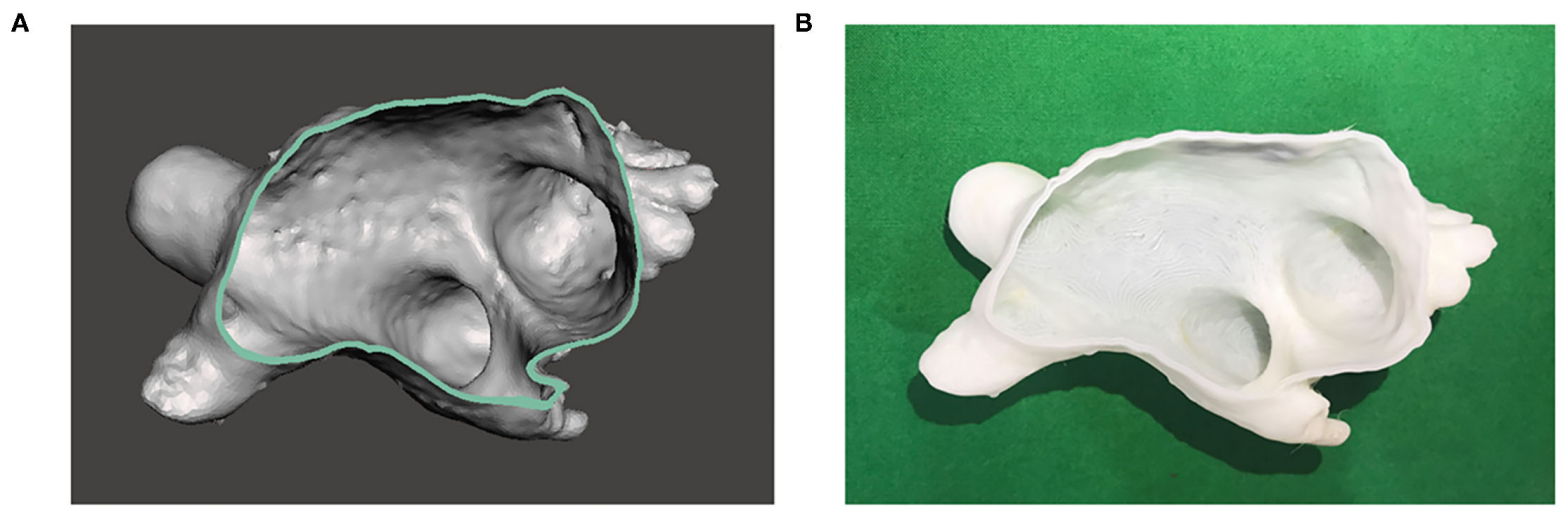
Three-dimensional (3D)-printed model of the left atrium (LA). (A) 3D image of the LA is reconstructed based on cardiac CT images. (B) 3D model of the LA is printed based on the image.
